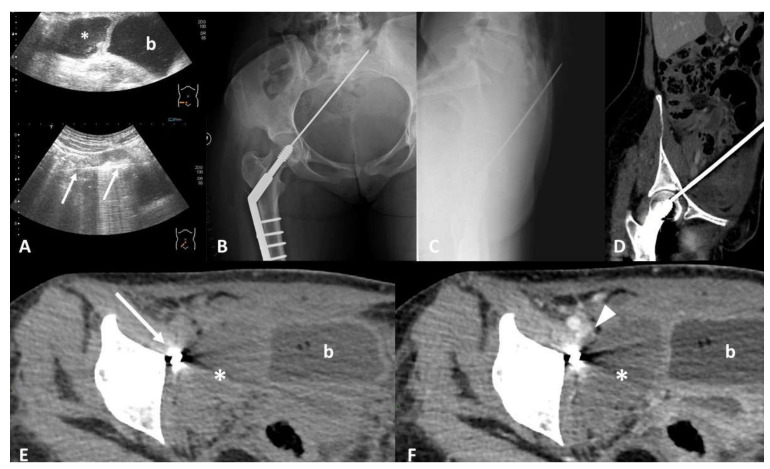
Device identification and recognition. Twenty-nine-year-old female with acute abdominal pain after surgical repair of a traumatic femoral neck fracture. Ultrasound scans (A) show a hypoechoic collection (*) in the right iliac fossa, close to the bladder (b), and a linear hyperechoic image with sonographic artifacts (arrows). Frontal (B) and lateral (C) radiographs show a sharp portion of the osteosynthesis passing through the pelvis, with the tip into the soft tissue of the anterior abdominal wall. Computed Tomography (CT) multiplanar reconstruction images better show the course of the misplaced device from the femoral head to the anterior abdominal wall (D). Non-enhanced CT scan (E) confirms a large collection (*) in the right iliac fossa, next to the misplaced device (arrow), characterized by hematic density. Contrast-enhanced CT images (F) better define the relationship between the collection (*) and the iliac vessels, showing a hypodense filling defect (arrowhead) inside the right external iliac vein (EIV), suggesting the diagnosis of a hematic collection due to iatrogenic injury of the EIV caused by the misplaced device.
